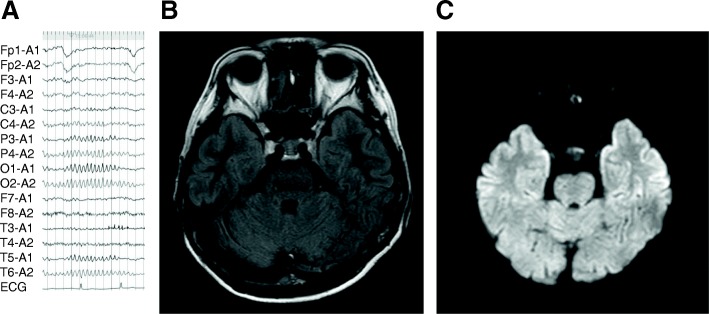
Imaging findings on admission with psychiatric symptoms after interferon-free therapy. Electroencephalogram showed 10–11 Hz with an irregular unsteady α wave dominant in the occipital lobe and without paroxysmal abnormality (a). Brain magnetic resonance imaging in fluid-attenuated inversion recovery (b) and diffusion-weighted imaging (c) revealed normal findings, and drug-induced encephalopathy was not developed
